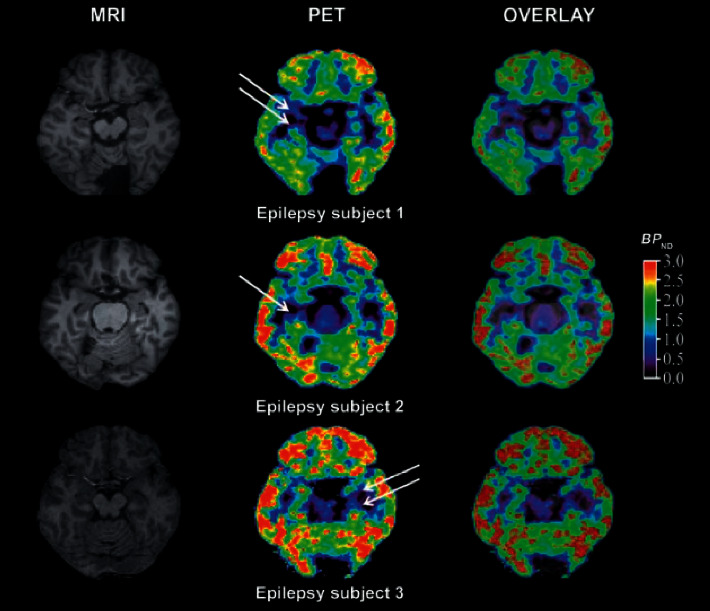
^18^F-FDG imaging of the bilateral prefrontal cortex showed slow advance and fast exit, and the total amount decreased.
